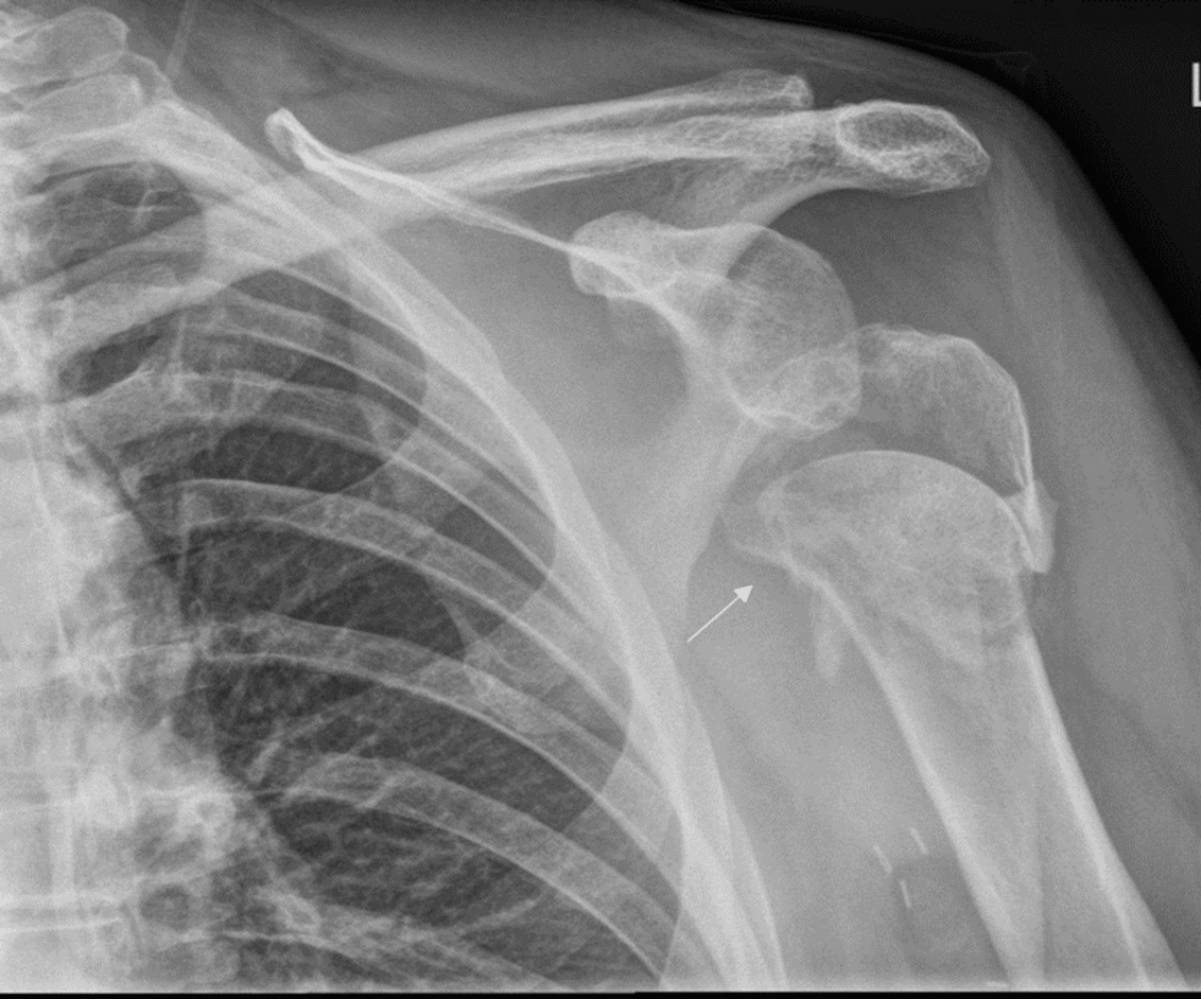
Preoperative anteroposterior radiograph of the left shoulder demonstrating a comminuted fracture-dislocation of the proximal humerus The white arrow indicates the fracture and dislocation.
